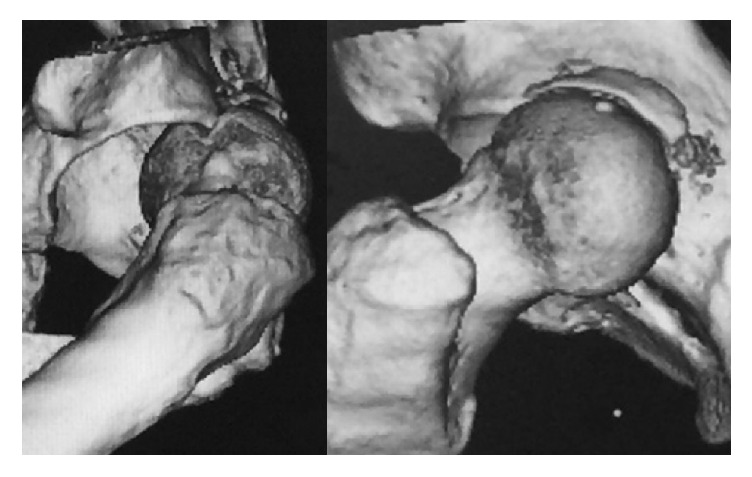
Computed tomography suggested a bone chip from the posterior wall of the acetabular roof and a depressed femoral head that cut into the posterior margin of the acetabular roof.
